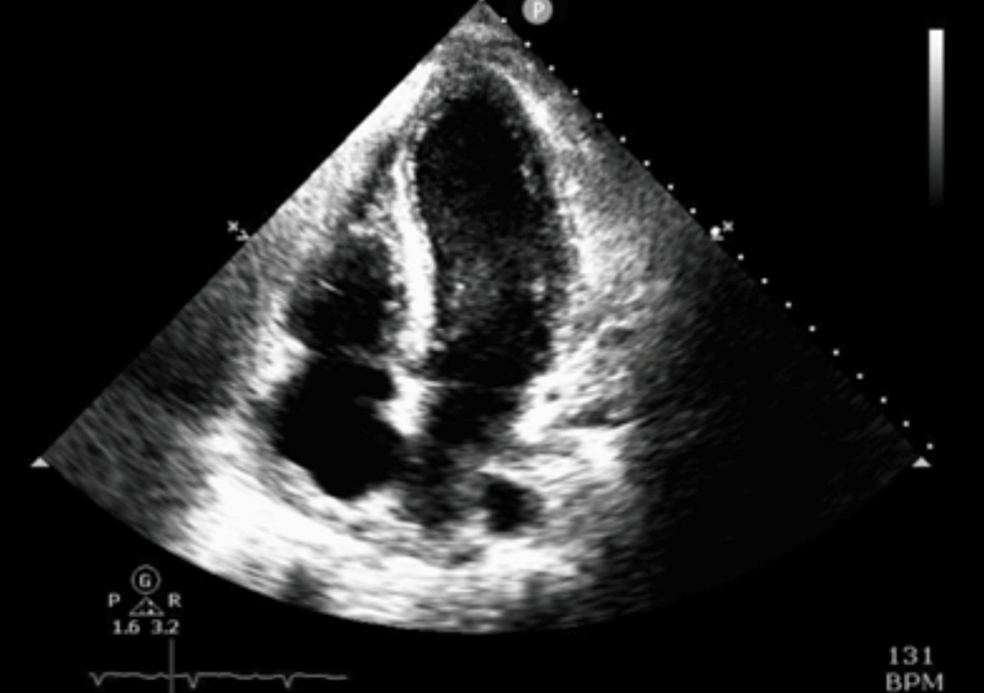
The apical four-chamber view shows complete resolution of pericardial effusion.
